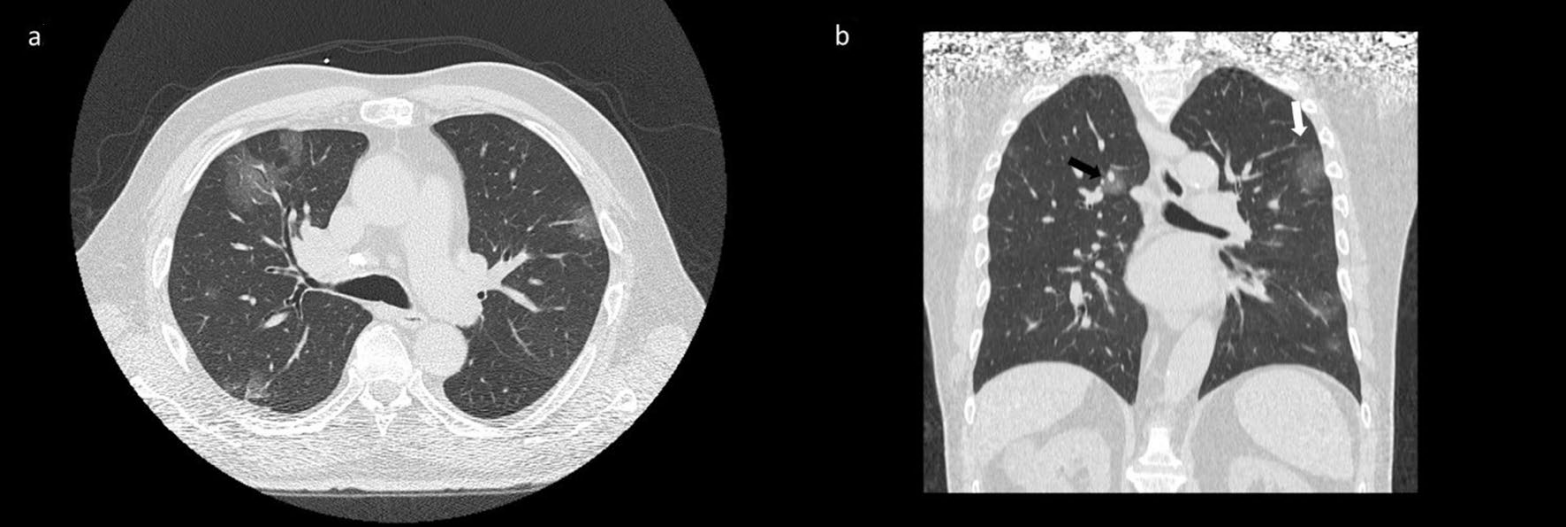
(a) Axial CT scan thought upper lobes shows bilateral areas of GGO with central (black arrow) and peripheral (white arrow) distribution as depicted in the CT coronal reformation (b).
